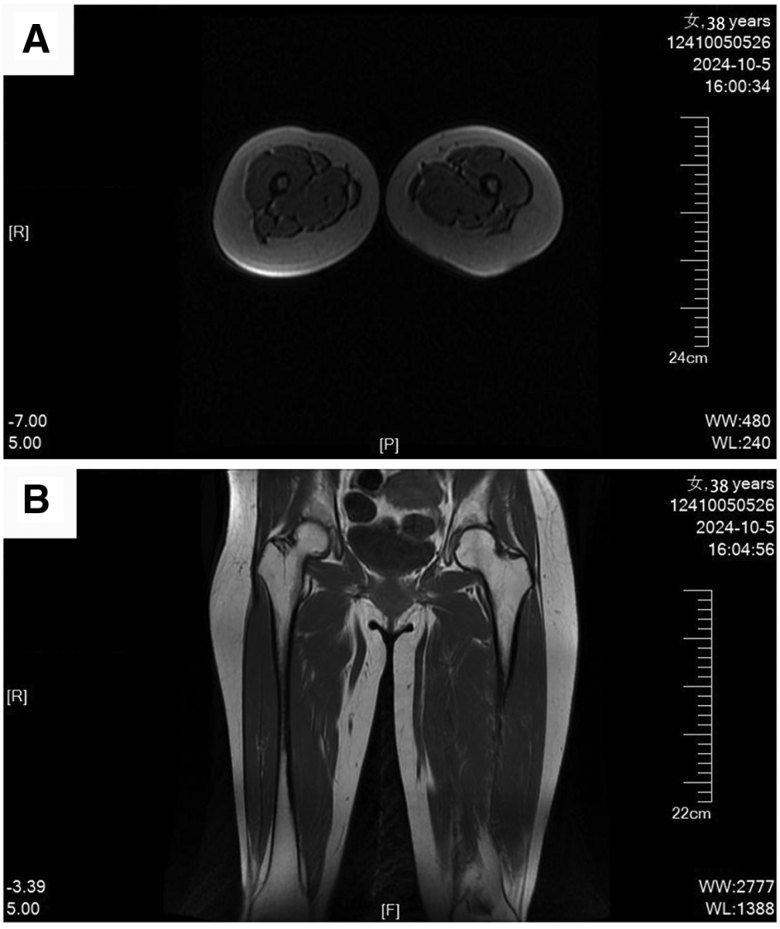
Results of plain MRI of both lower extremities were normal; no abnormal signal shadows indicating skin or muscle swelling were observed. (A) Transverse plane. (B) Coronal plane. MRI = magnetic resonance imaging.
